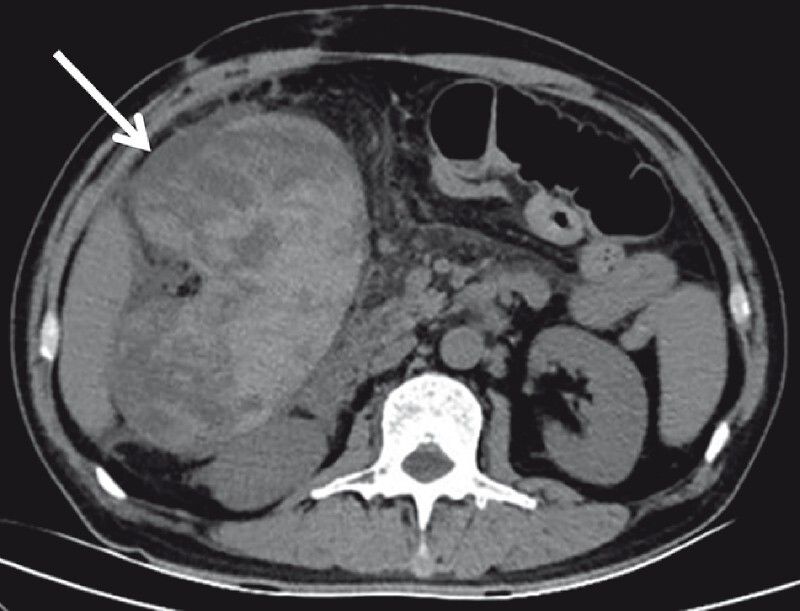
A preoperative abdominal computed tomography (CT) indicating complete colonic obstruction with high-density contents consistent with bleeding.
